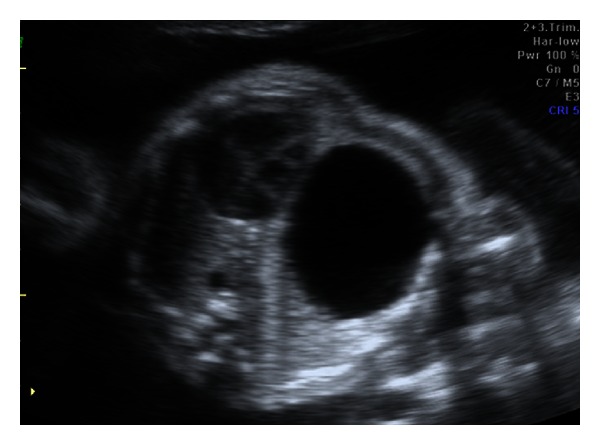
Prenatal ultrasound of axial plane showing a great cyst image in the left hemithorax compression of the normal left lung tissue and contralateral mediastinal shift.
